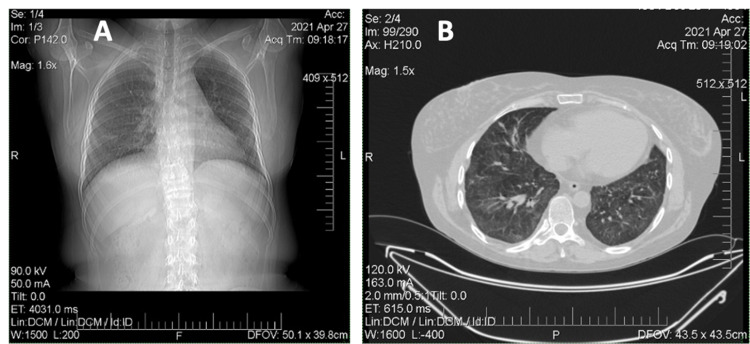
A, B: Multislice computed tomography of the chest (April 2021). A: Vertical tomography slices. B: Horizontal tomography slices.  In the parenchyma of both lungs, mainly in the hilar and posterior regions, there is a thickening of the interlobular interstitium with fibrous layers. The lumen of the bronchi is not expanded. In the previous study, an expansion of the subsegmental bronchi of the lower lobes was noted.
